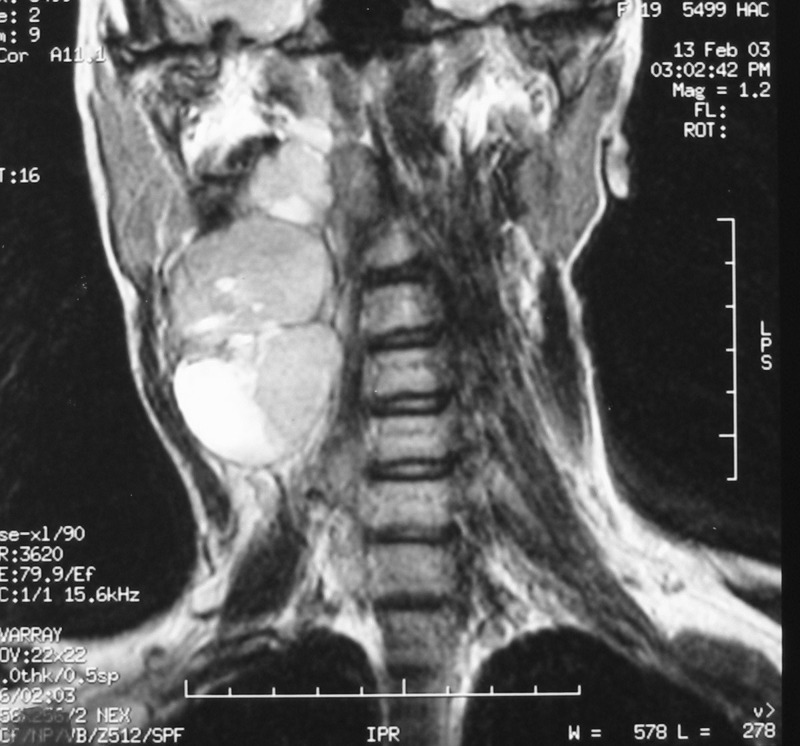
Coronal T2-weighted MR image showing a laterocevical tumor with heterogeneous signal.
